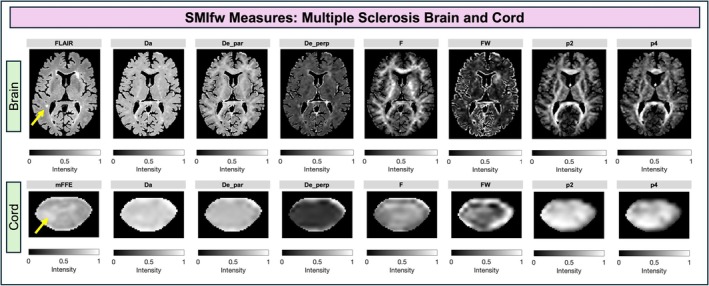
All measures captured by in the SMIfw diffusion model for one pwRRMS (58.5‐year‐old male) in the brain and SC. The FLAIR and mFFE images were normalized between 0 and 1 for visualization and included as anatomical references, with lesions identified via the yellow arrow. For diffusion metrics, intensity values within brain and cord regions were independently normalized by their respective 99th percentile values to scale diffusion maps between 0 and 1 for visualization.
